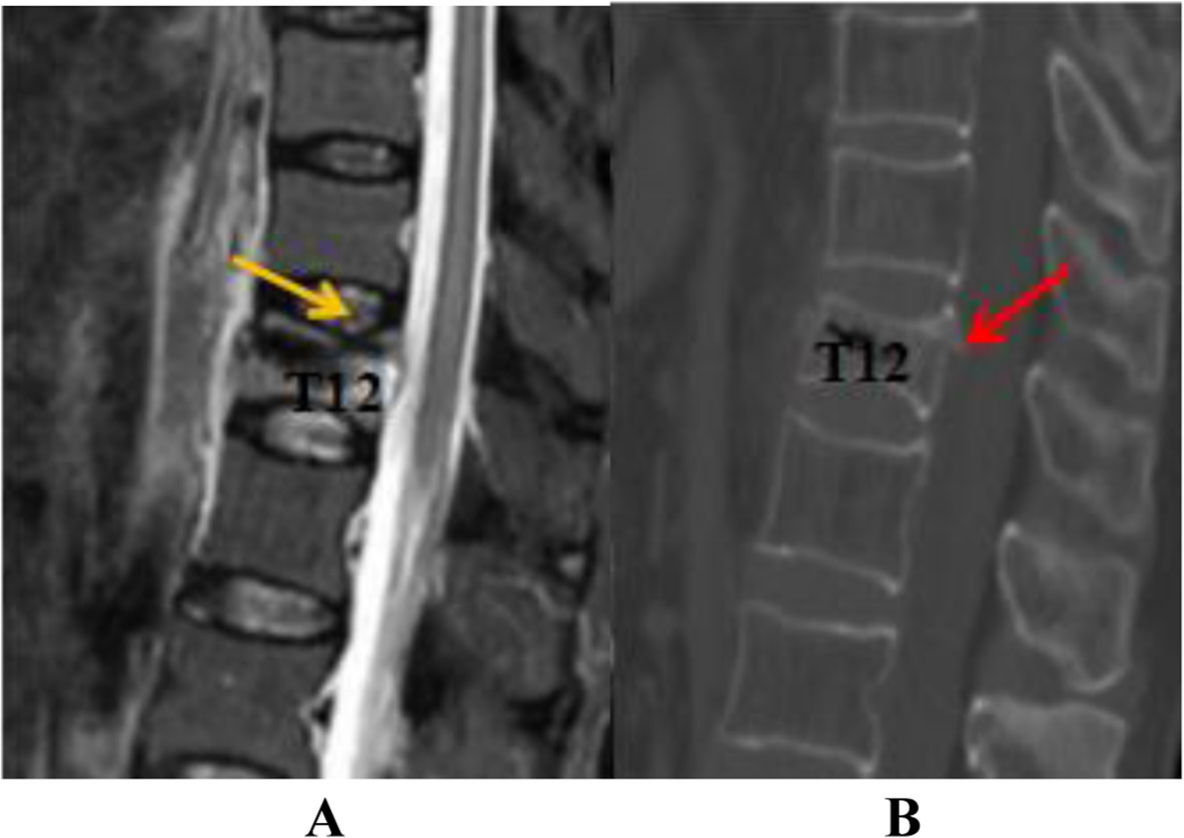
A 74-year-old woman with OVF at level T12. Panel a is an MRI STIR image. The yellow arrow indicates a diffuse high signal in the IVD on the cranial of the fractured vertebral body and a fracture of the upper endplate of the vertebral body. Figure b is a CT image of the fractured vertebral body. The red arrow indicates that the upper endplate of the T12 vertebral body is fractured, and the endplate collapse is accompanied by a fracture of the posterior vertebral body. This is a grade 4 EDC injury as delineated in this study
